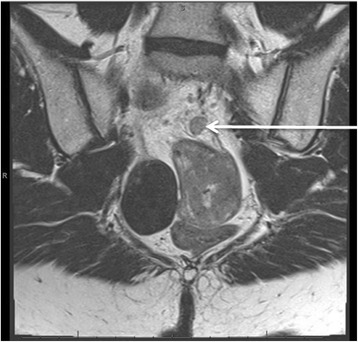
Preoperative MRI demonstrating the largest (0.9 cm) of multiple perirectal lymph nodes
